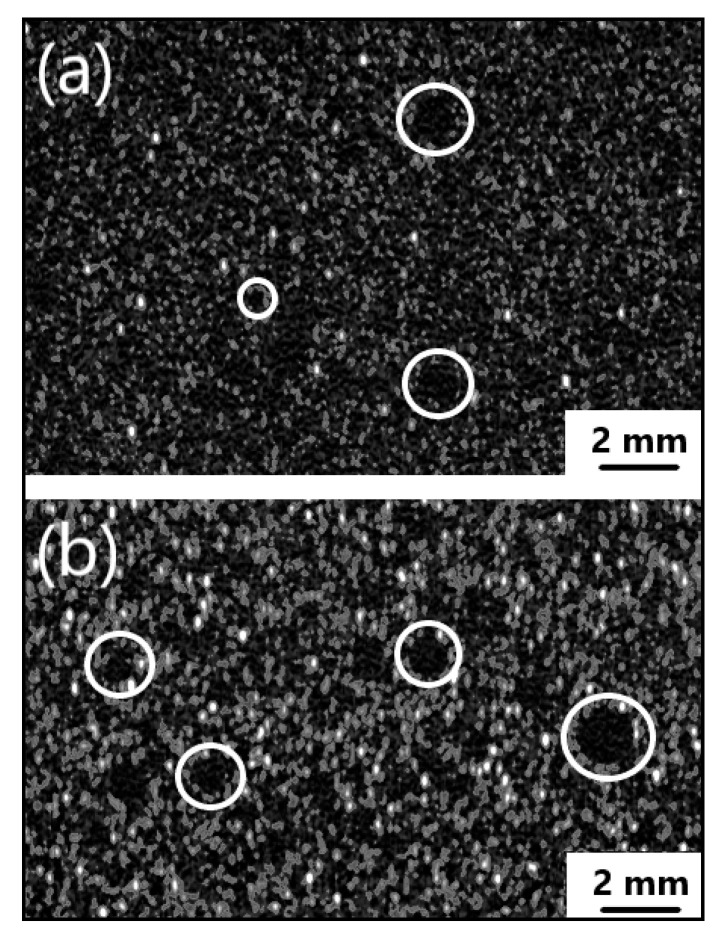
CT images (plane cross-sections) for RPUF with (a) 5 wt.% of filler; (b) 10 wt.% of filler.
